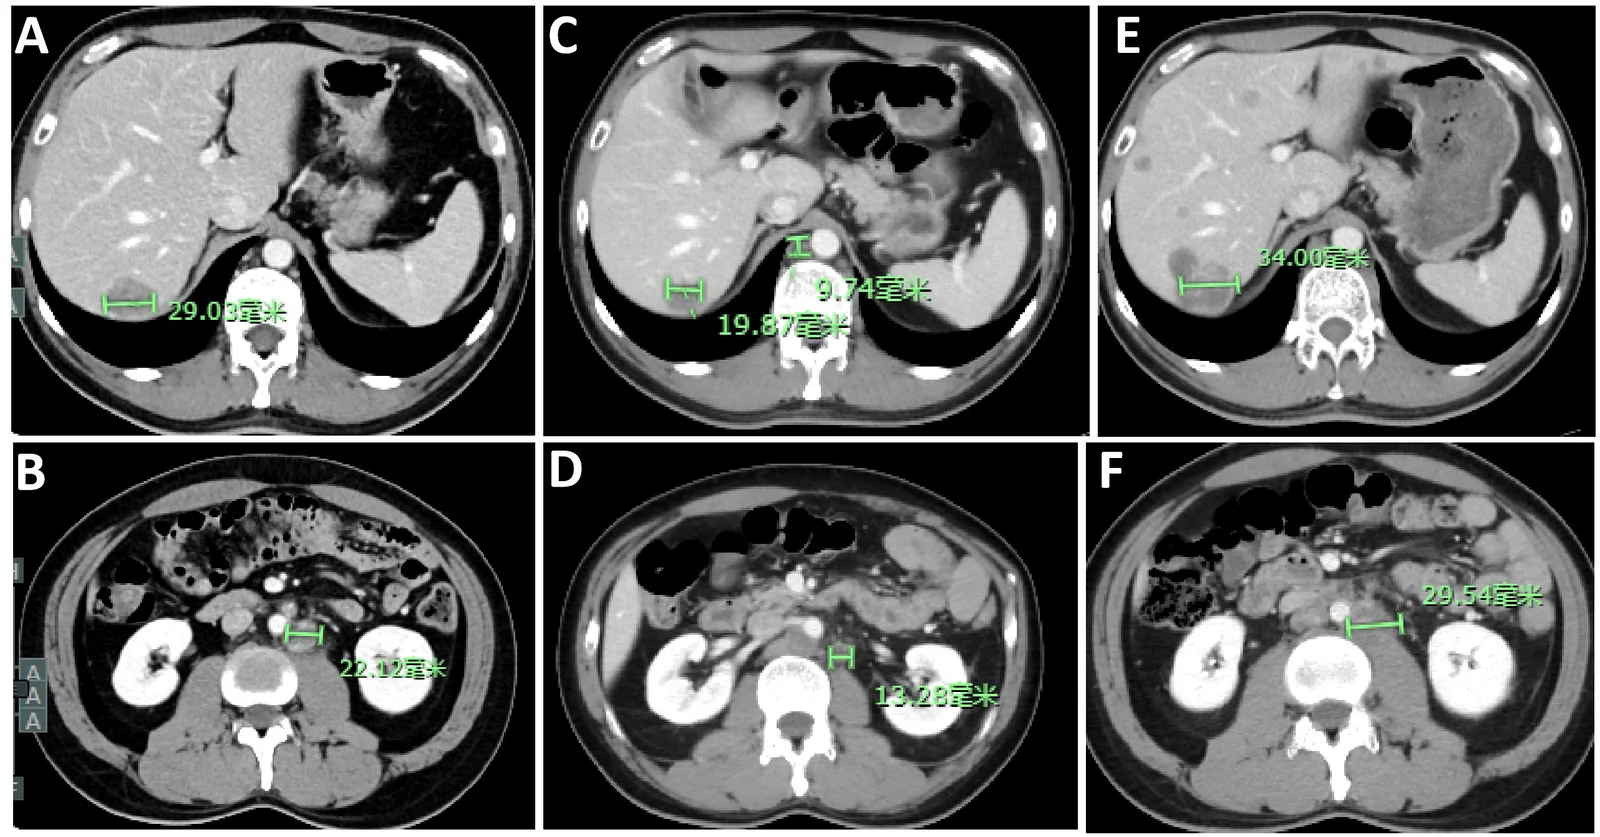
Serial CT images during fifth-line sintilimab plus surufatinib therapy. (A, C) Baseline (April 14, 2023). Sum of target lesions: 5.1 cm. (B, D) Best response (October 11, 2023). Sum of target lesions reduced to 2.2 cm (−56.9%); per RECIST, best overall response: partial response (PR). (E, F) Progression (January 24, 2024). Target lesions enlarged to 6.3 cm (+23.5% vs. baseline); per RECIST, progressive disease (PD). Scan dates are shown in each panel. All images are at comparable anatomical levels.
